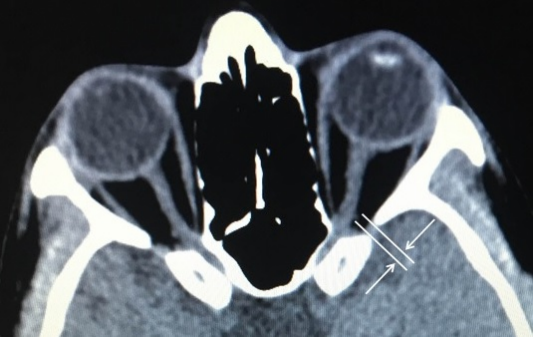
CT scan on the horizontal plane; the arrows indicate the length to measure.
